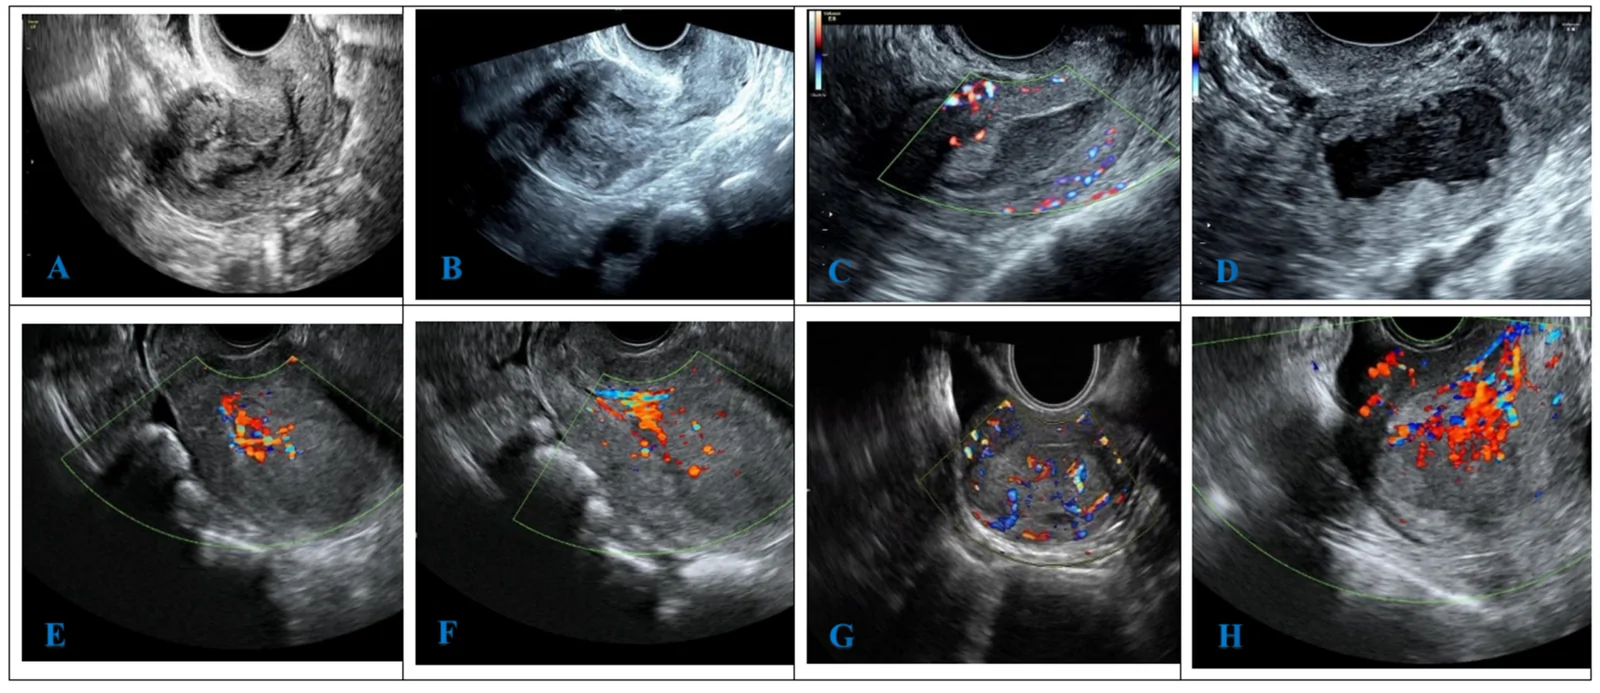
Images of malignant IETA ultrasonographic features. (A) Heterogeneous with irregular cysts and endometrial–myometrial junction: “interrupted”; (B) Endometrial midline appearance: “not defined” and endometrial–myometrial junction: “not defined”; (C) Intracavitary fluid: “ground glass”; (D) Intracavitary fluid: “mixed” echogenicity; (E,F) Vascular pattern: Multiple vessels (focal origin); (G) Vascular pattern: Multiple vessels (multifocal origin); Color score: moderate amount of color/moderate vascularity (3 points); (H) Color score: abundant color/abundant vascularity (4 points).
